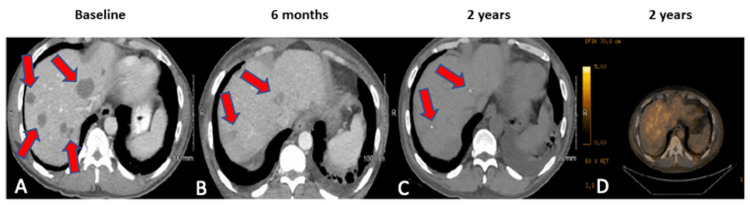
Serial CT scans The serial scans show progressive tumor shrinkage with eventual complete remission. (A) CT performed at diagnosis. (B) CT performed 6 months after the initiation of immunotherapeutic treatment. (C) Two-year follow-up CT scan performed without contrast as the patient developed nephropathy. (D) PET-CT-confirmed continued complete response at the 2-year mark.
